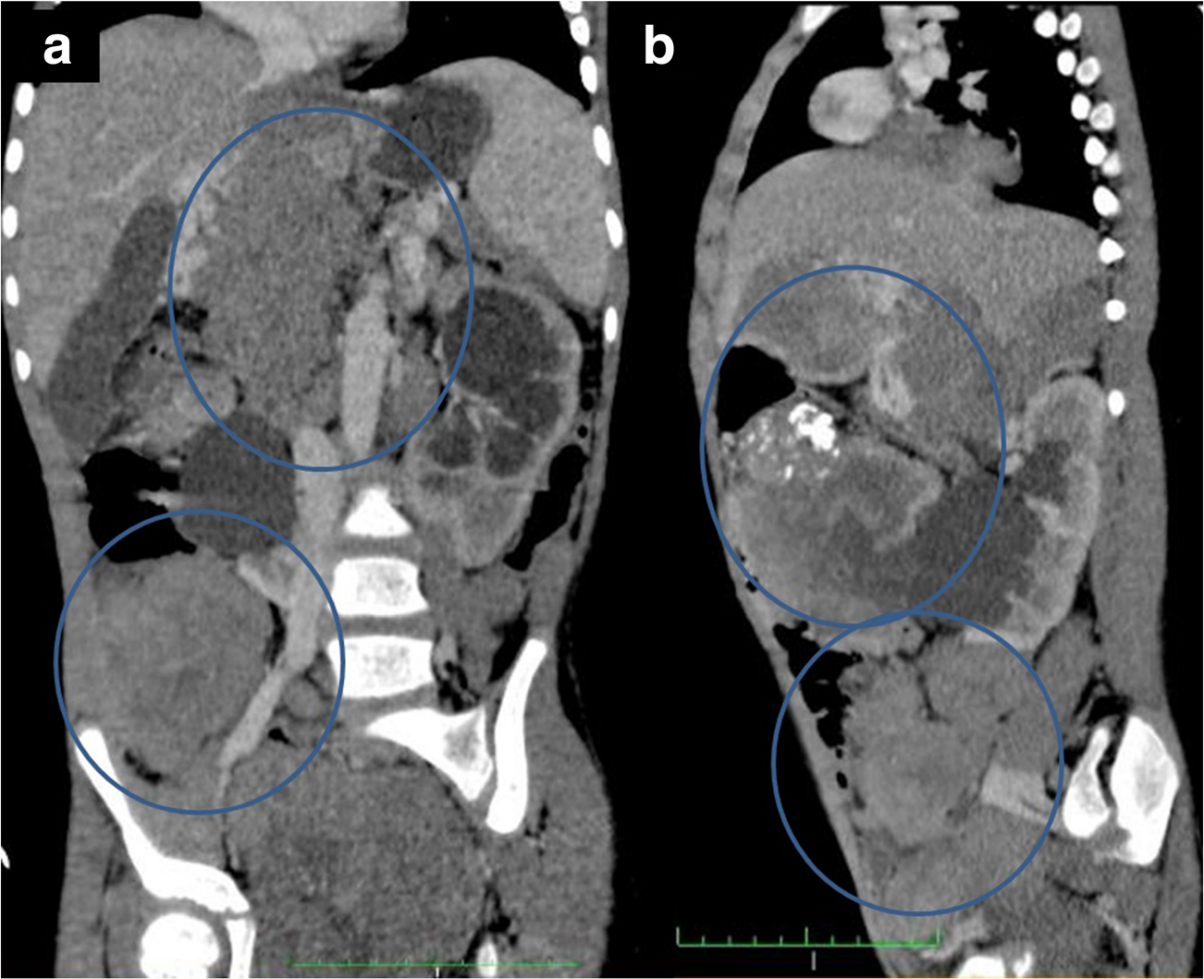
Abdominal and pelvic CT: coronal (a) and sagittal multiplanar reconstruction (b)—pathologic soft tissue mass in abdomen and pelvis (multifocal PNET) with calcifications, left kidney hydronephrosis
